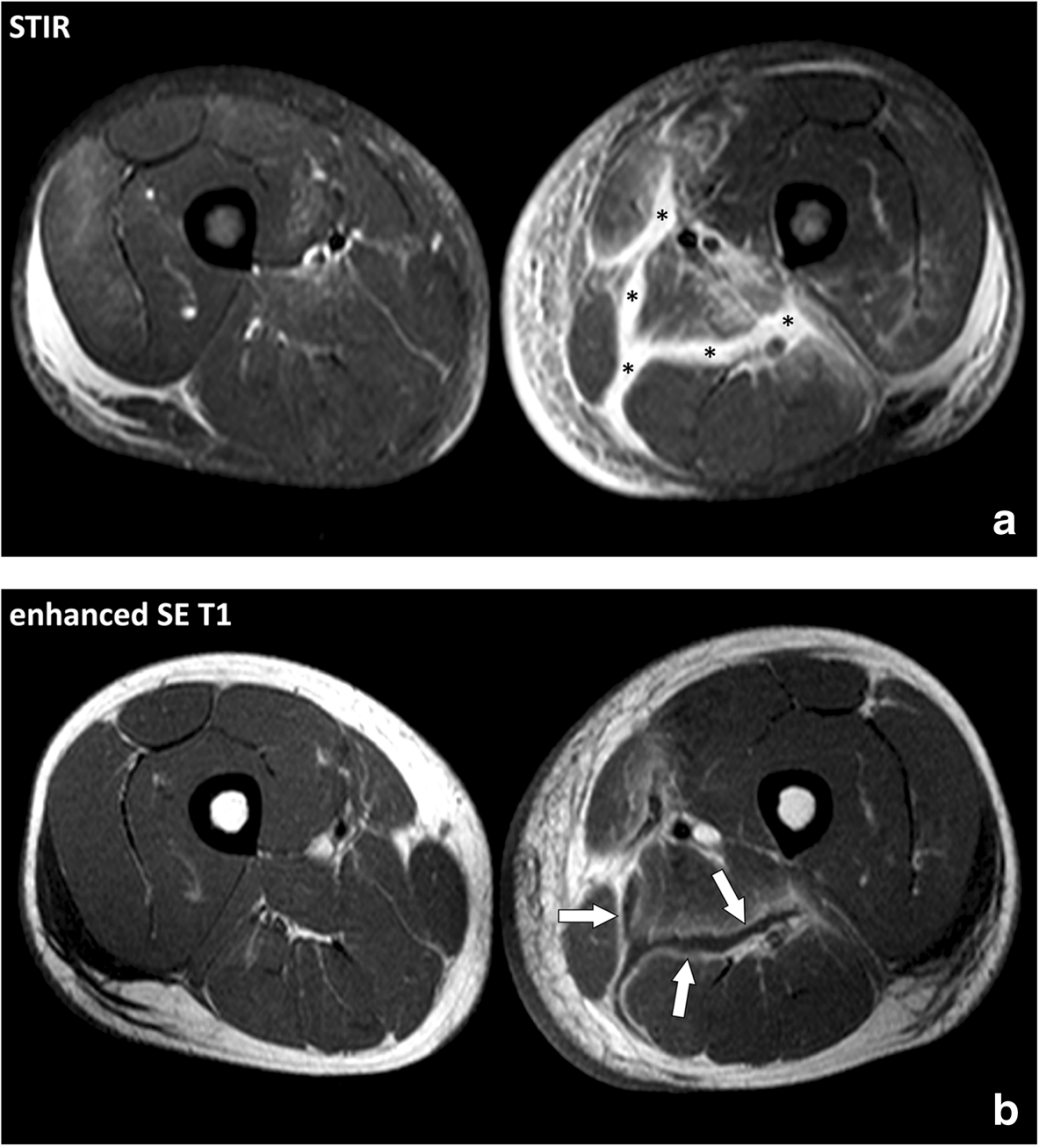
Axial (a) STIR and (b) contrast-enhanced SE T1w images of the thighs of a 34-year-old male with *Staphylococcus aureus* septicemia and necrotizing fasciitis of the left thigh. MRI demonstrates thickening of the deep intermuscular fasciae of the posteromedial compartment of the thigh with fluid-like signal on the STIR image (asterisks) and enhancement adjacent to the necrotic fasciae after contrast material injection (arrows)
